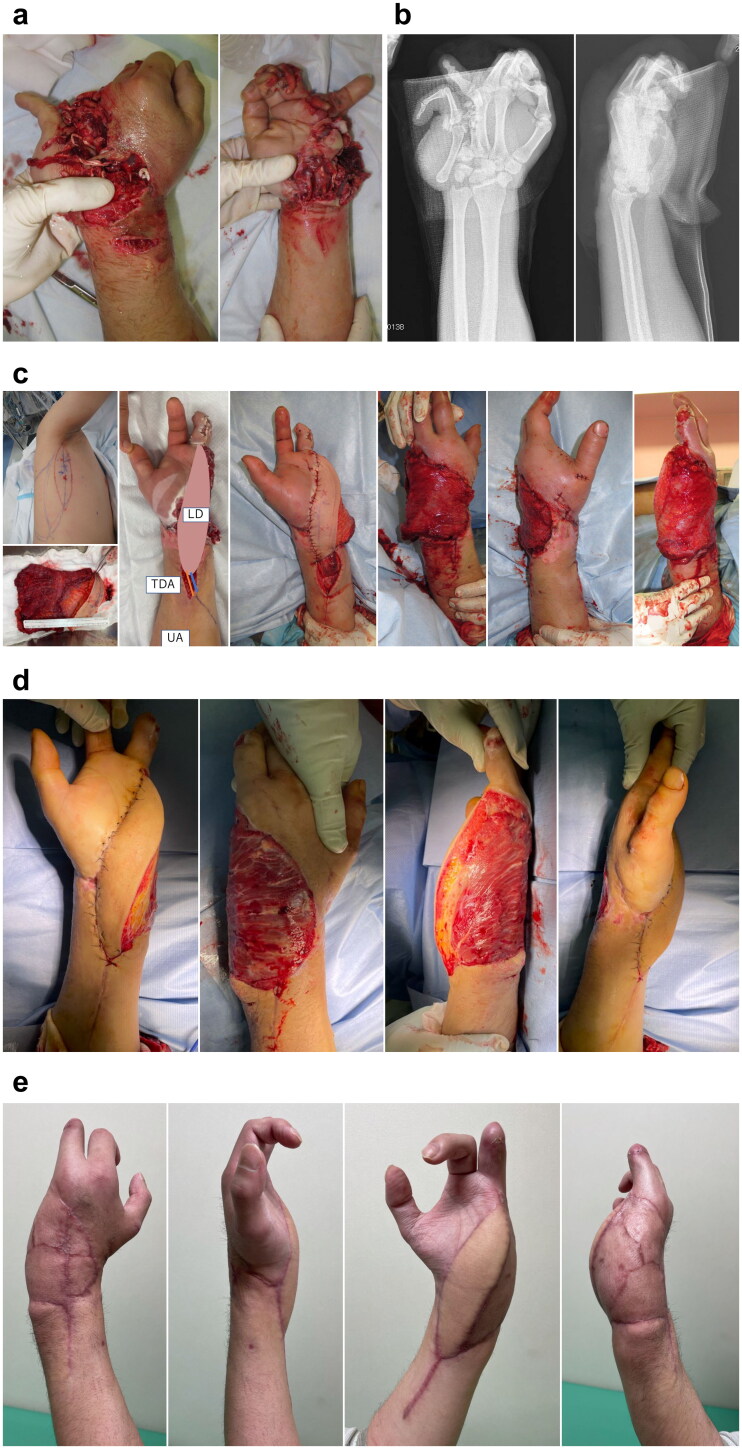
(A) Appearance on his arrival. (B) Radiographic examination on his arrival. (C) Free LD flap was performed at 1 week after injury. The recipient artery was sutured end-to-end to the ulnar artery. (D) After the third tangential excision, (E) Findings at 3 months after free LD flap. LD: latissimus dorsi.
